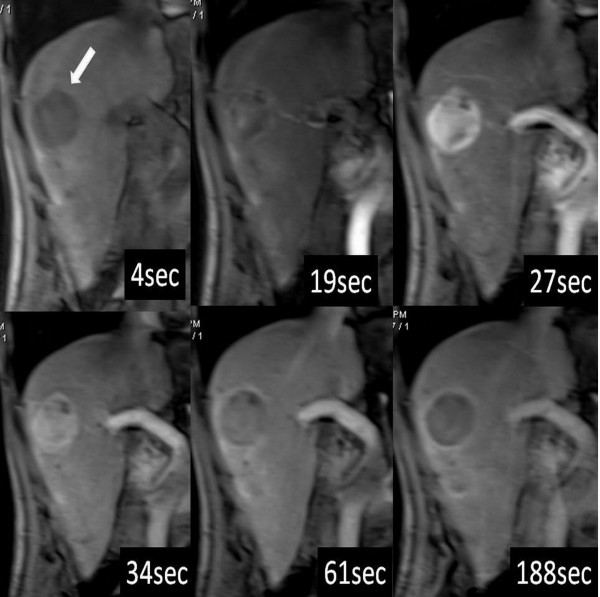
Perfusion MR images of the liver in a 49-year-old male patient with HCC, which showed classic enhancement features of hyper-vascularity at arterial phase and washout at portal venous phase and delayed phase by selected time points from 32 measurements.
